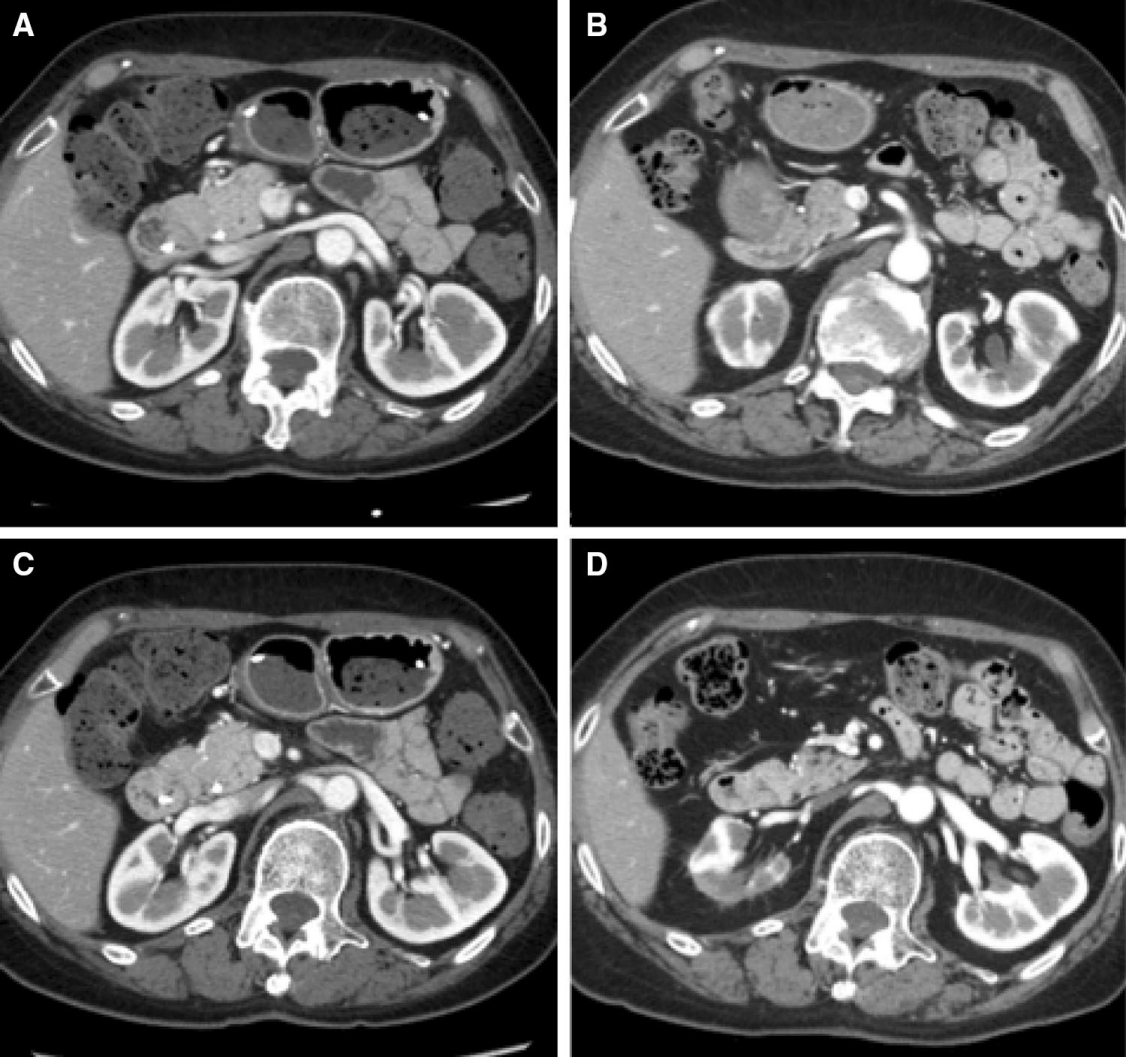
CE-CT images before glucocorticoid therapy for type 1 autoimmune pancreatitis (a, c) and on admission (b, d). CE-CT on admission revealed multiple low-density lesions in the bilateral kidneys and marked atrophy limited to the right kidney. The left kidney showed only partial atrophy (b, d)
